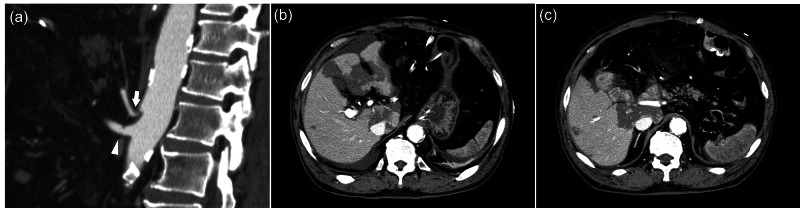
Contrast-enhanced CT on postoperative day 1 (a) Sagittal view of the arteries (arrow, “hook”-shaped celiac artery (CA); arrowhead, superior mesenteric artery). Note that the superior mesenteric artery is also shifted cranially compared with that of the preoperative CT. (b) Segmental liver infarction can be seen. In the hilar area of the liver, the hepatic artery shows decreased blood flow. (c) Splenic infarction is also confirmed.
